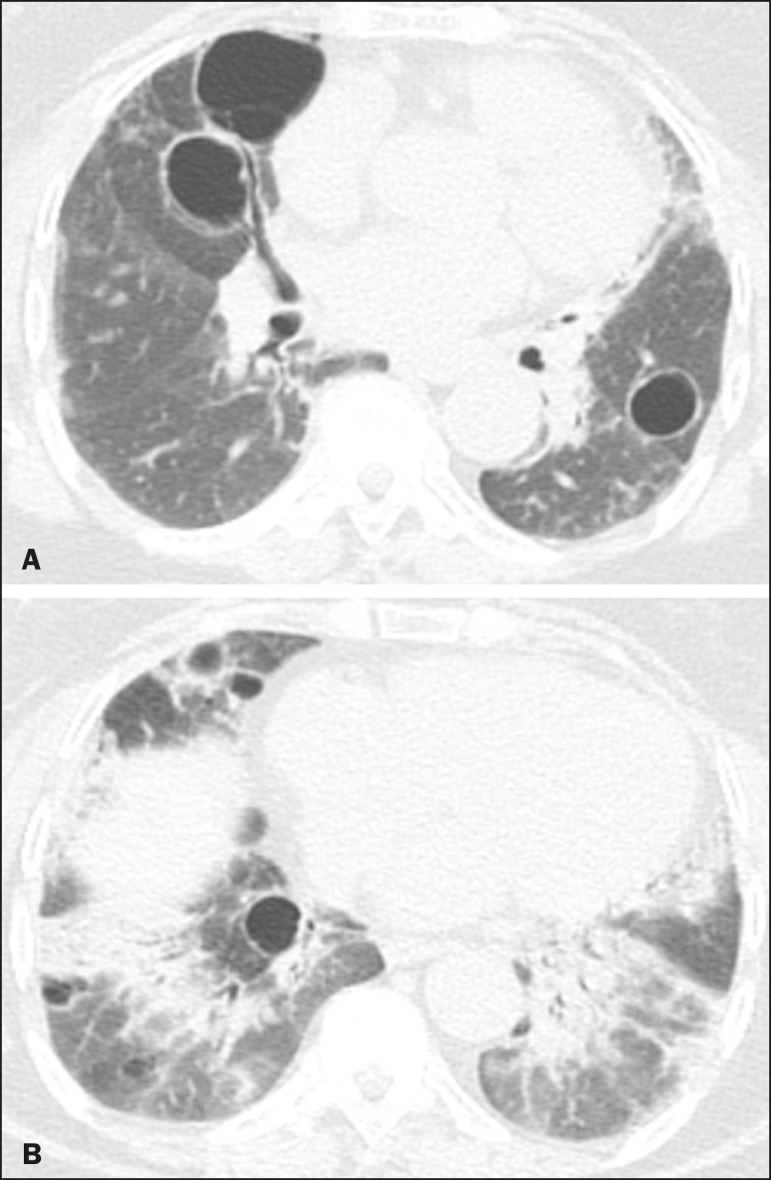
A 64-year-old man with idiopathic LIP. Chest CT scans show multiple cysts with a random and bilateral distribution associated with ill-defined ground-glass opacities and consolidations.
